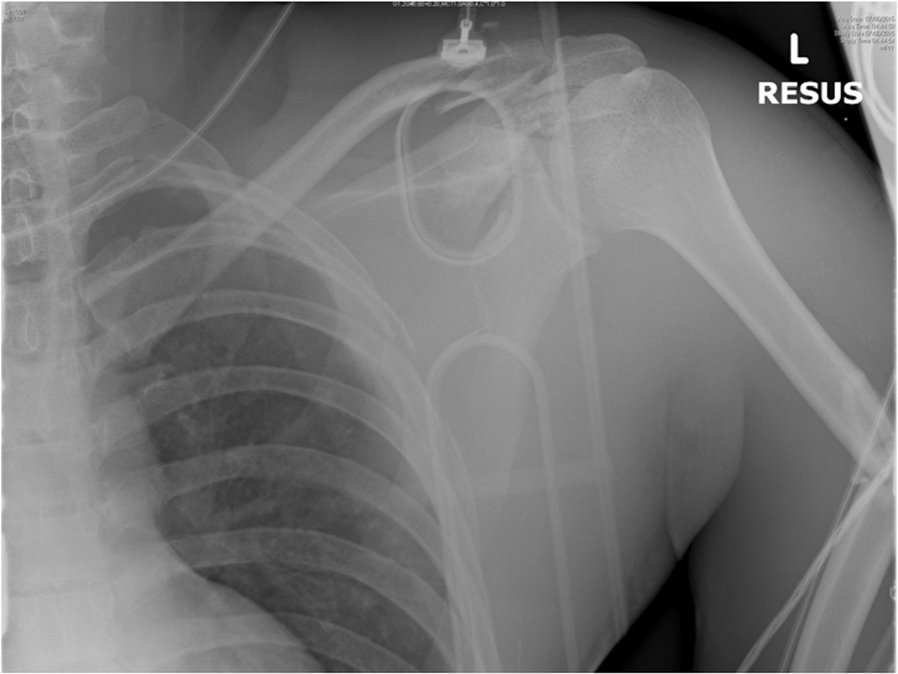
Plain X-ray of the left shoulder. Plain X-ray of the left shoulder showing fractures of the left scapula body, left humerus, left distal clavicle, left 3–8th ribs, and left chest wall soft tissue swelling
